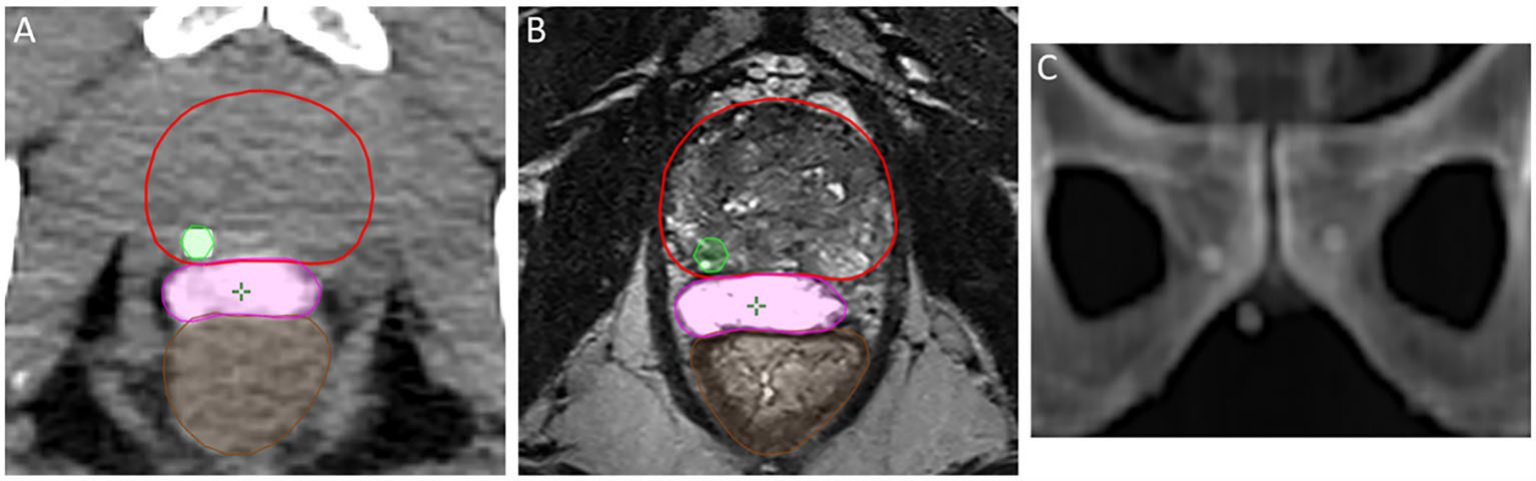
Proper placement of the hydrogel spacer (magenta) achieving posterior rectal displacement, and location of gold fiducial marker in the prostate parenchyma (green) on axial non-contrast CT (A) and axial T2-weighted MRI (B). Multi-planar spacing of fiducial markers as seen on DRR (C).
